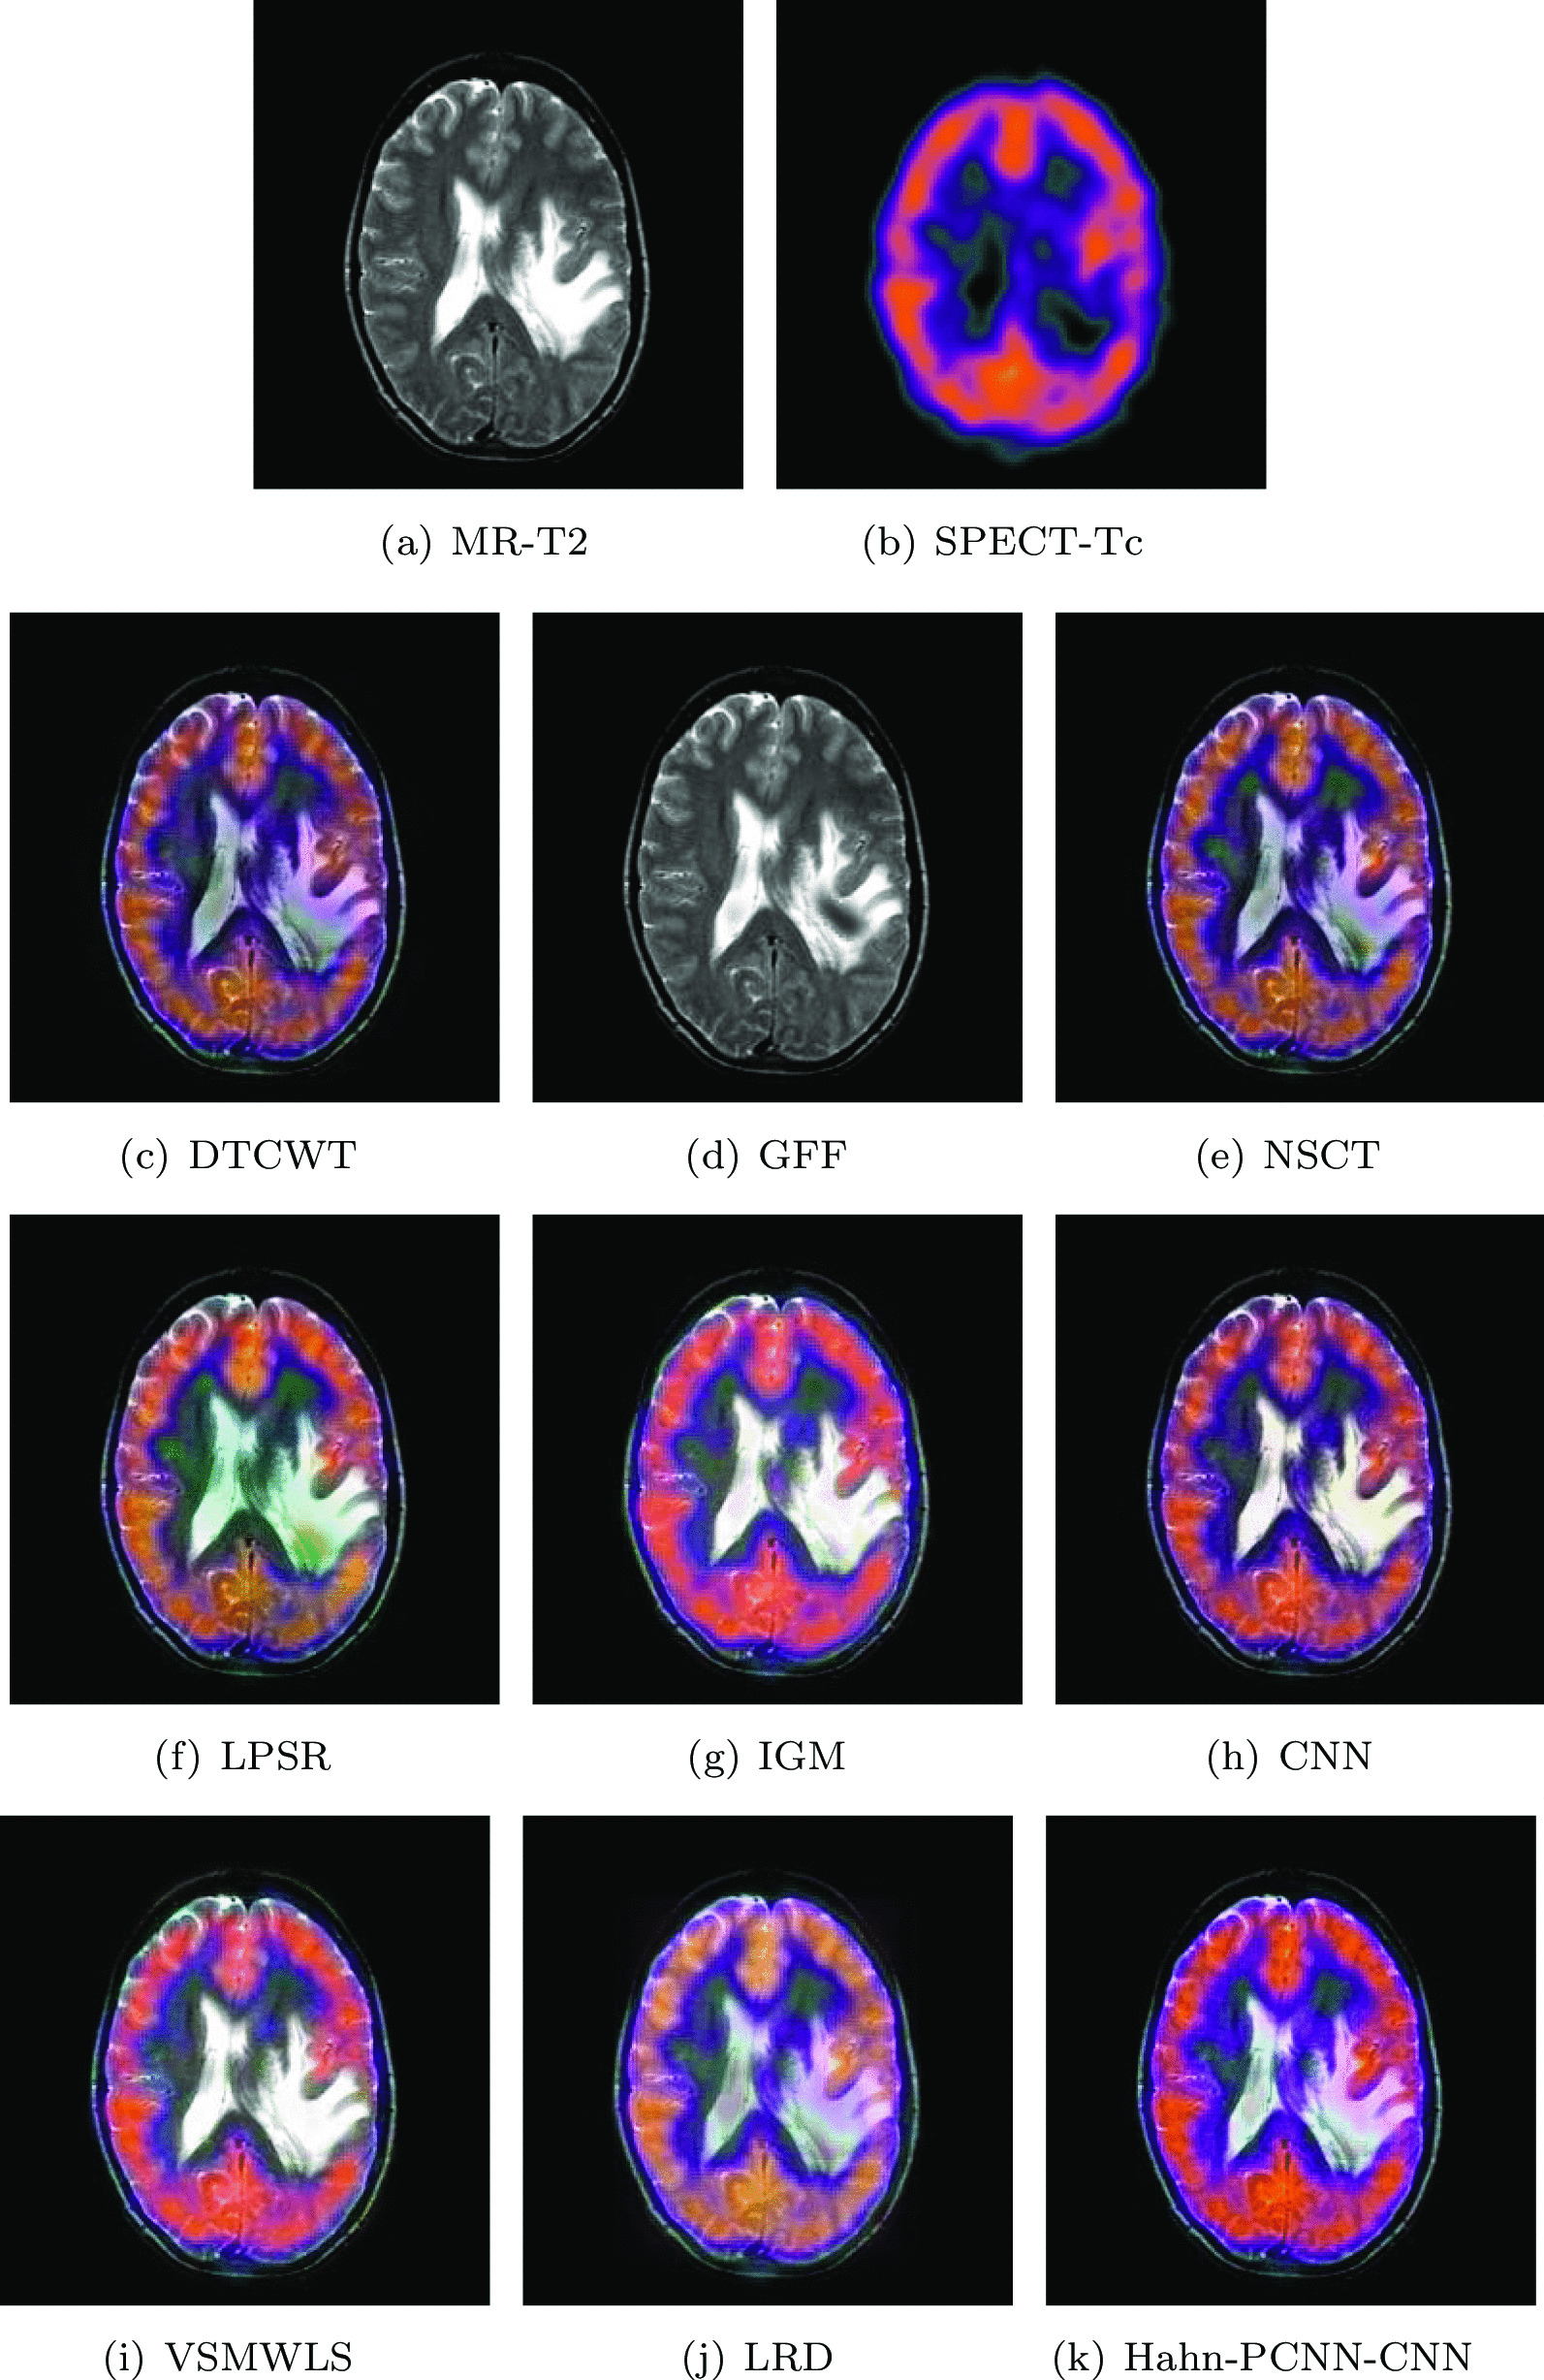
The first set of fused MRI-SPECT images from 9 methods on metastatic bronchogenic carcinoma
